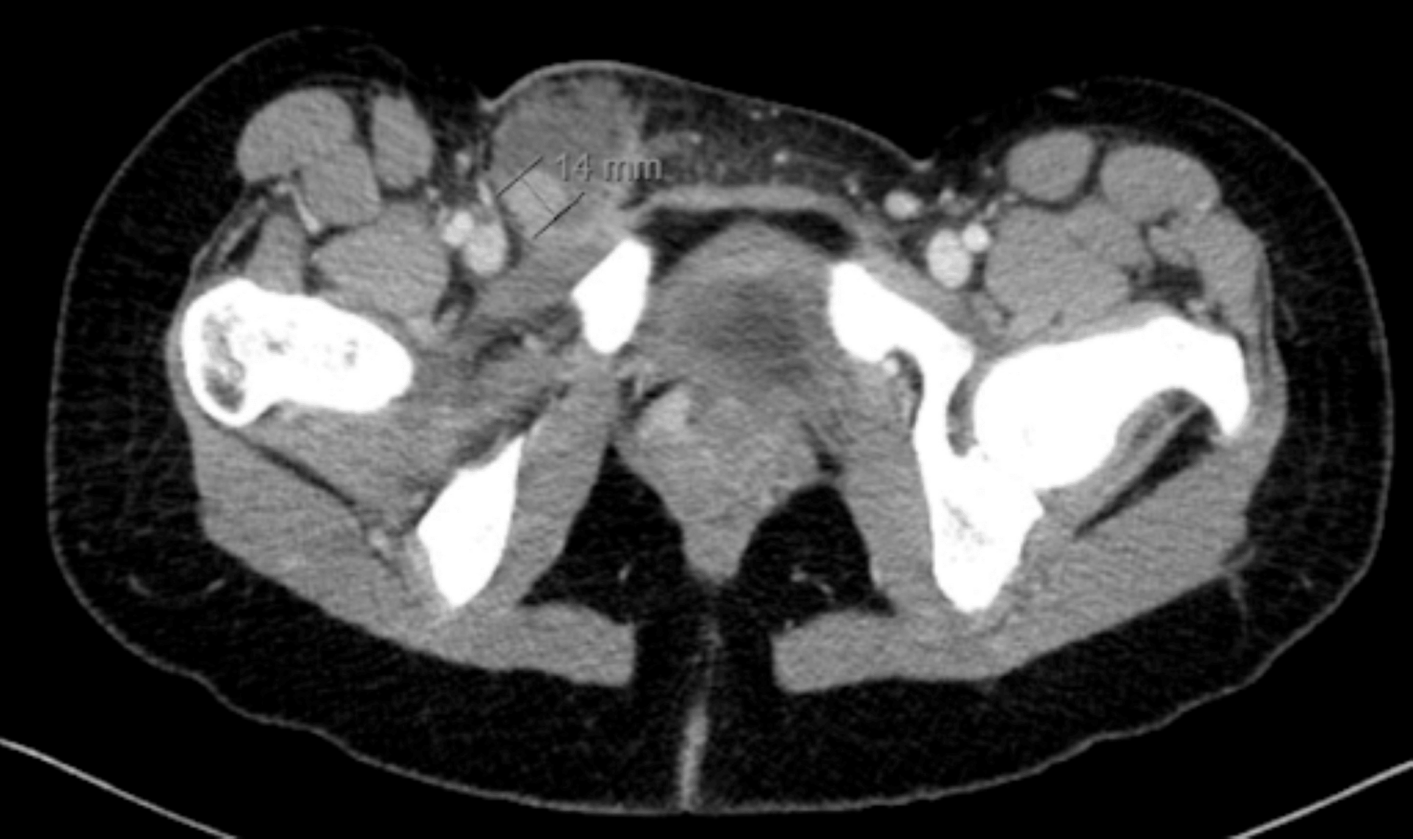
CT pelvis, static image Findings suggest a pseudoaneurysm with a surrounding hematoma. No obvious signs of localized fat stranding.
